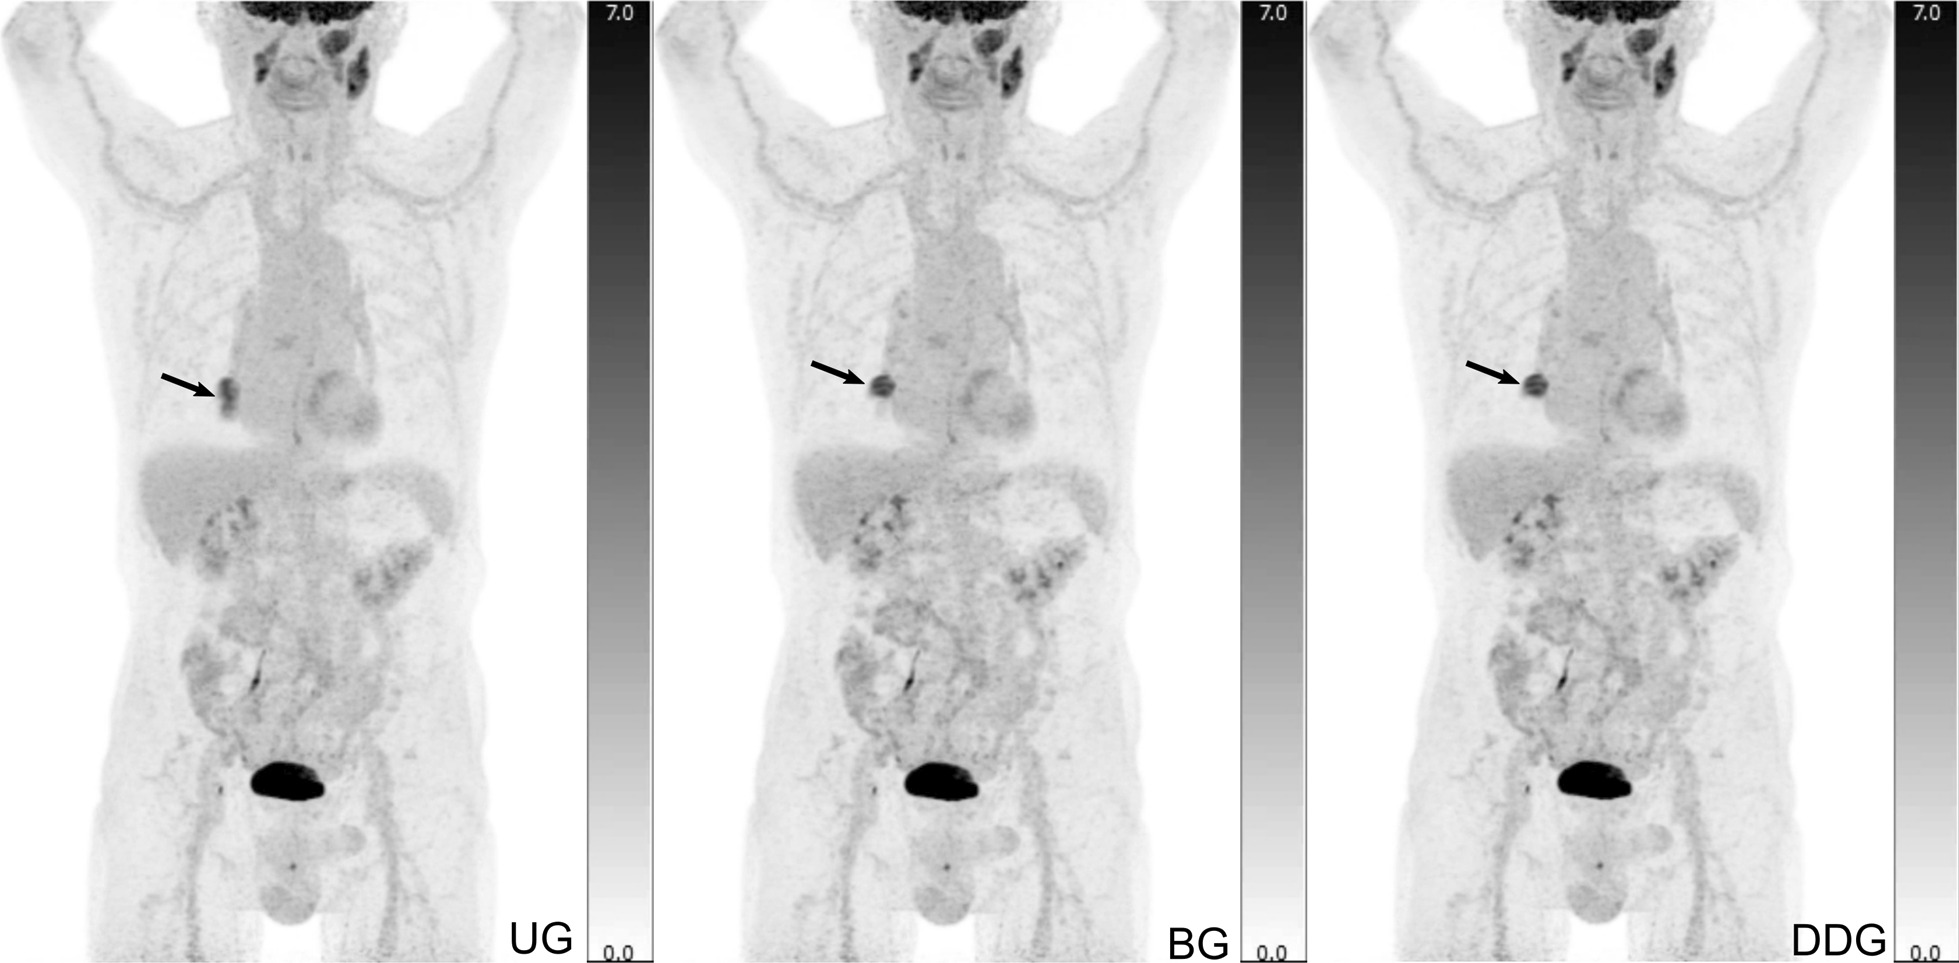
A 79-year-old male patient with lung cancer displaying a classic motion artefact. The ungated image (UG) appears to have a double contouring of the primary tumour (arrows), whereas the two motion compensated images (BG, DDG) clearly reveal a single lesion with sharper contours, smaller metabolic volume and increase in SUV values
